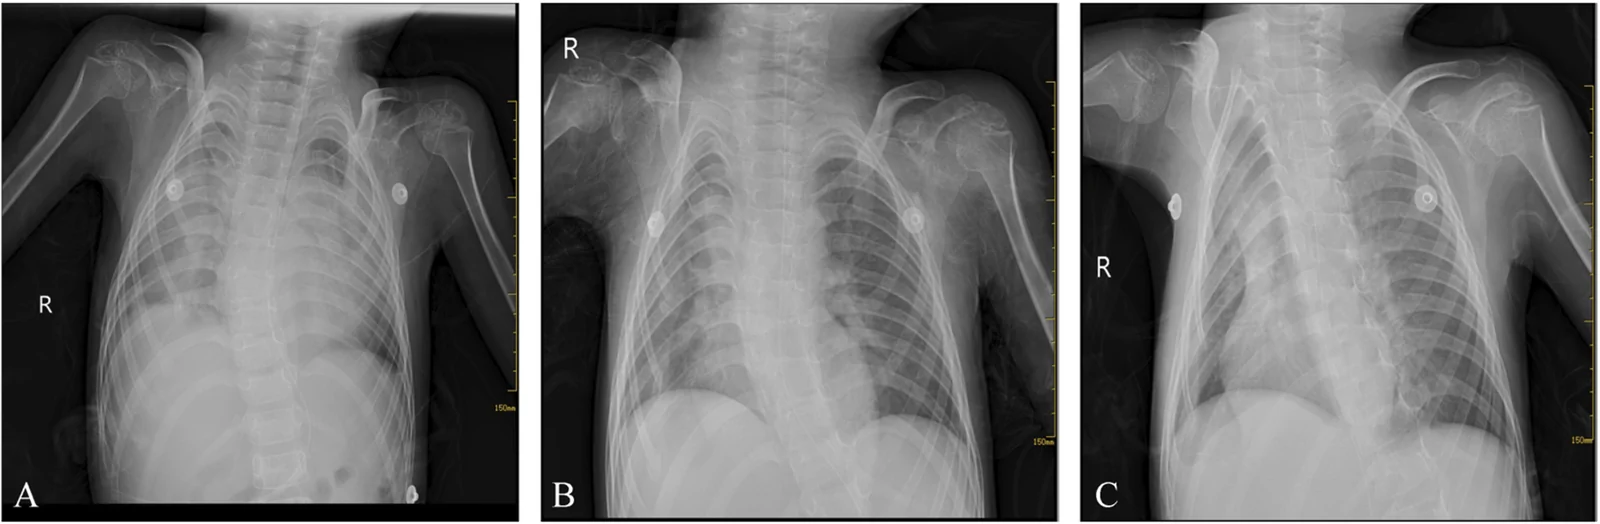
Chest radiographs showing progressive resolution of pulmonary infection. (A) Before SUL-DUR therapy, showing bilateral pulmonary infiltration. (B) Three days after initiation of SUL-DUR therapy, with noticeable improvement. (C) Ten days after initiation, showing substantial resolution of the infiltrates.
